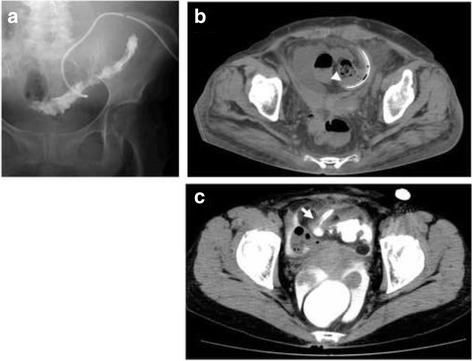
a Contrast catheterography showed accumulation of the contrast agents in the sigmoid colon. b The bowel perforation of PD catheter (arrowhead) was seen on computed tomography scan. c Computed tomography scan with contrast agents into the catheter (arrow) showed accumulation of the contrast agents in the colon and rectum
